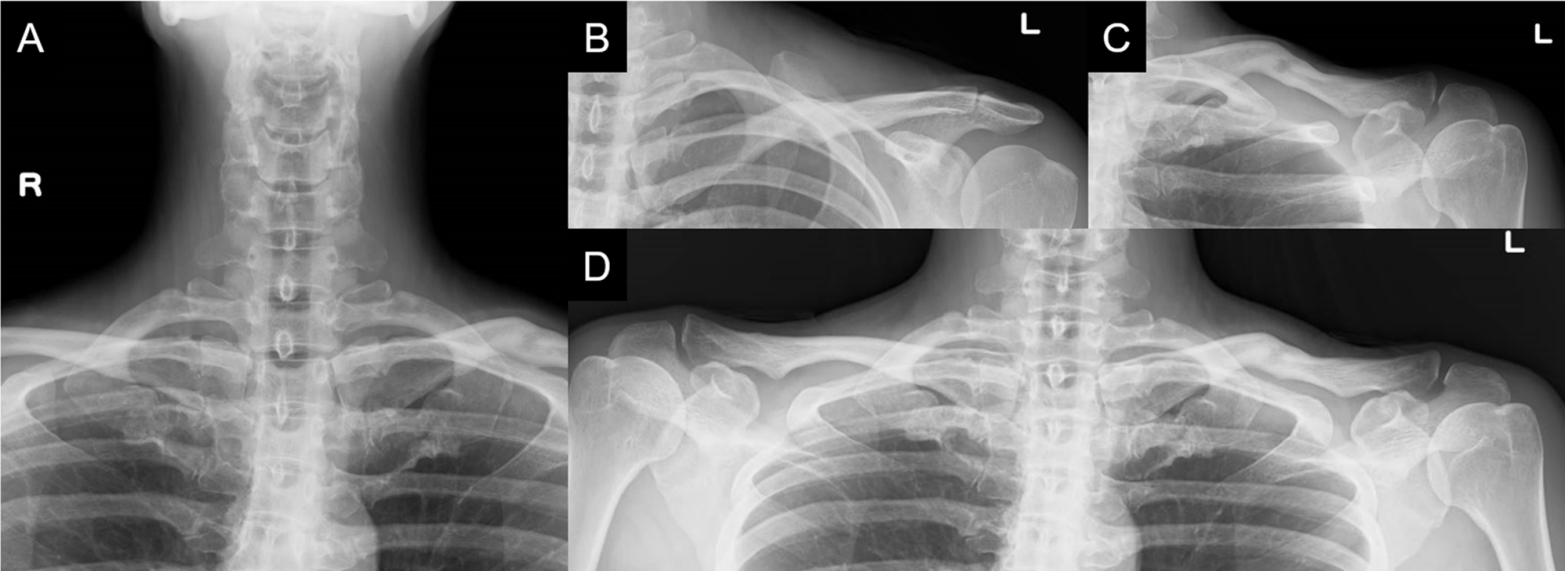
A radiograph of the cervical spine showing no cervical ribs or abnormal rib deformities (A). Radiographs of the clavicle showing malunion of the left clavicular midshaft fracture (B, C, and D)
